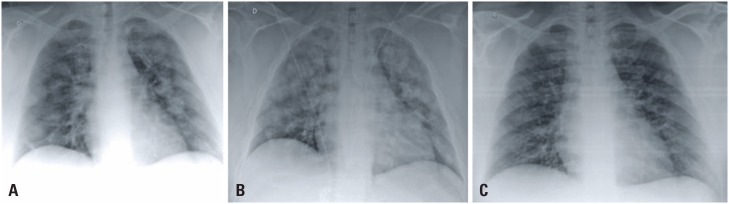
Bedside chest radiography at hospital admission (A), on day 2 (B) and day 10 (C) of hospitalization showed diffuse opacities in both lungs. Many of these opacities are seen to have nodular configuration that were more evident on the 2nd day of hospitalization (B)
